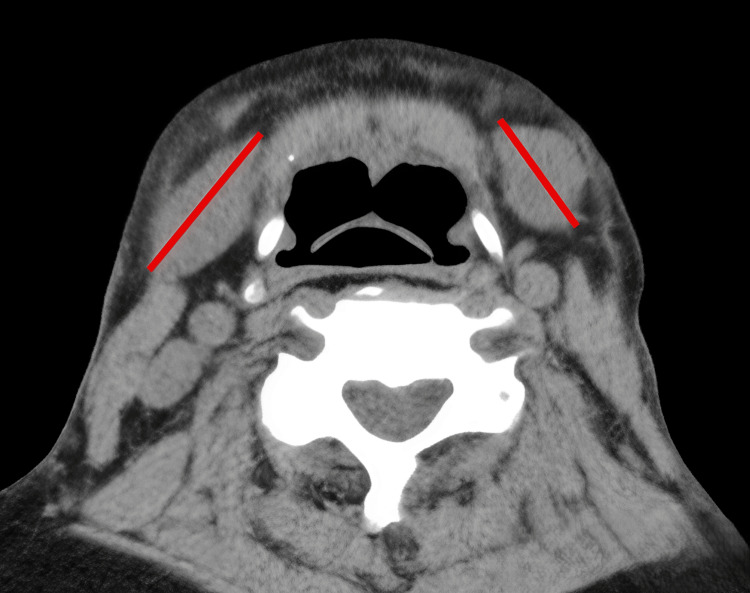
Submandibular gland reduction Axial CT image showing the proposed zone of partial submandibular gland reduction (incision line shown in red), intended to improve cervicomandibular definition and reduce bulk beneath the jawline.
